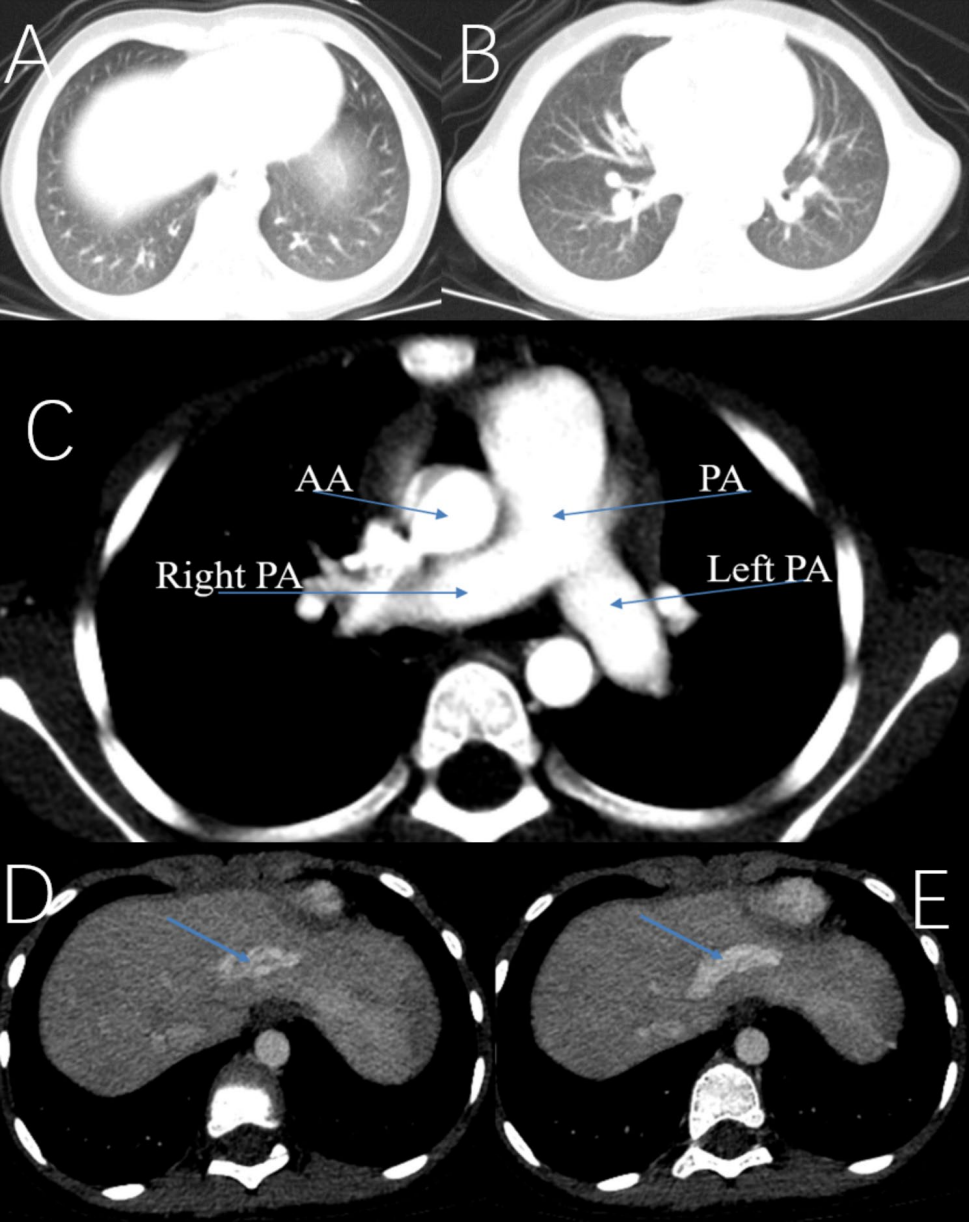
Contrast enhanced lung CT with vascular three-dimensional reconstruction revealed increased pulmonary vascular bundles and bronchovascular bundles (A, B), increased diameter of the pulmonary artery (PA), left PA right PA and ascending aorta (AA) (C), and left hepatic venous-left portal venous shunt (D, E)
